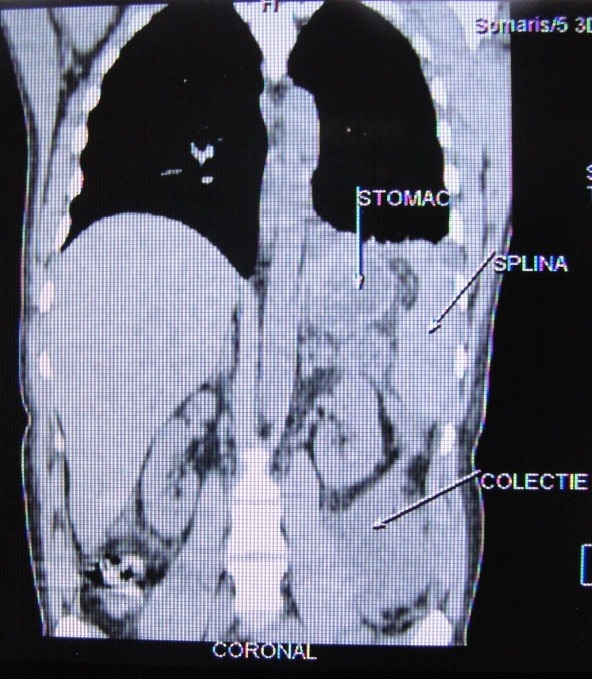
CT scan showing retroperitoneal necrotic extensions
